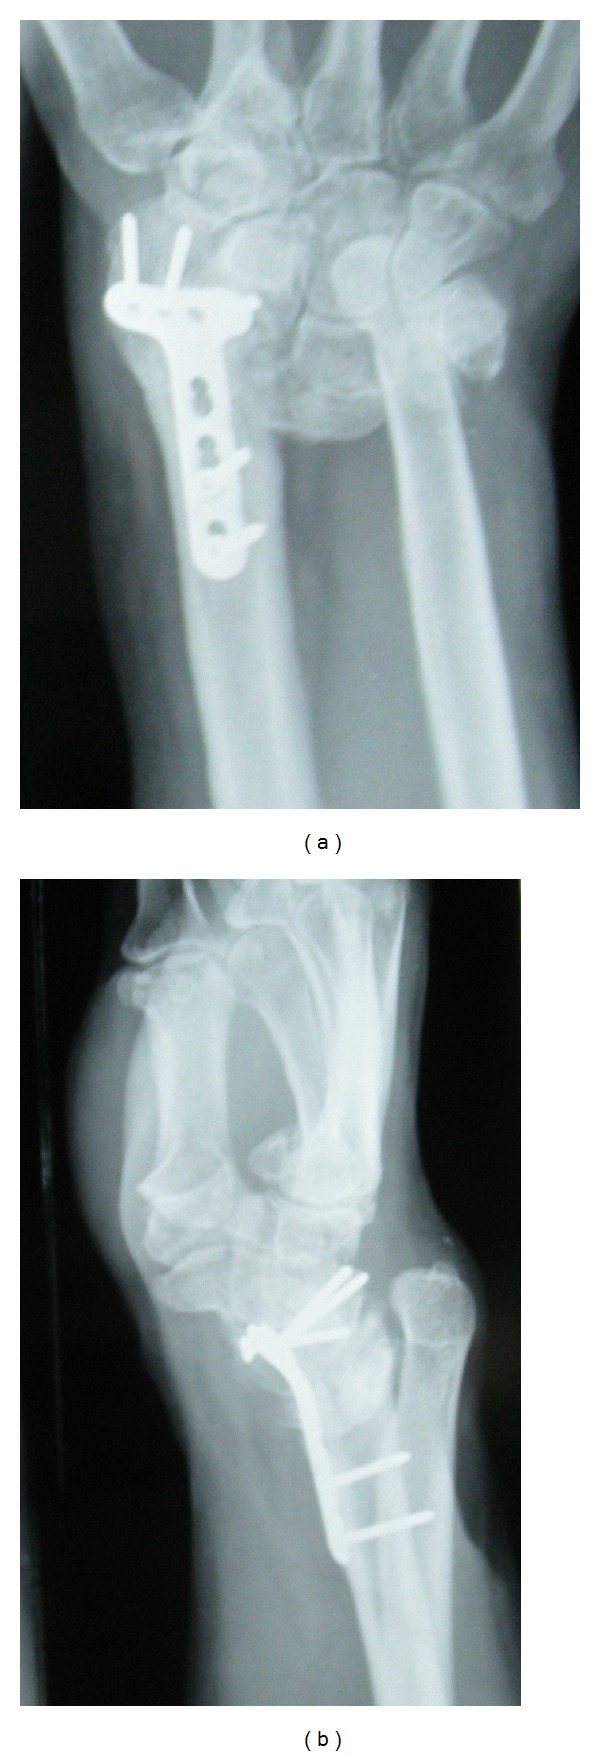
(a), (b) Radiographs at follow-up visit of an AO type C3 fracture of the right wrist which was fixed with volar locking plate. Though we were able to attain adequate reduction for the distal radius, the associated DRUJ (distal radioulnar joint) injury was missed by us. These radiographs show loss of reduction with dorsal subluxation of the carpus and DRUJ disruption.
